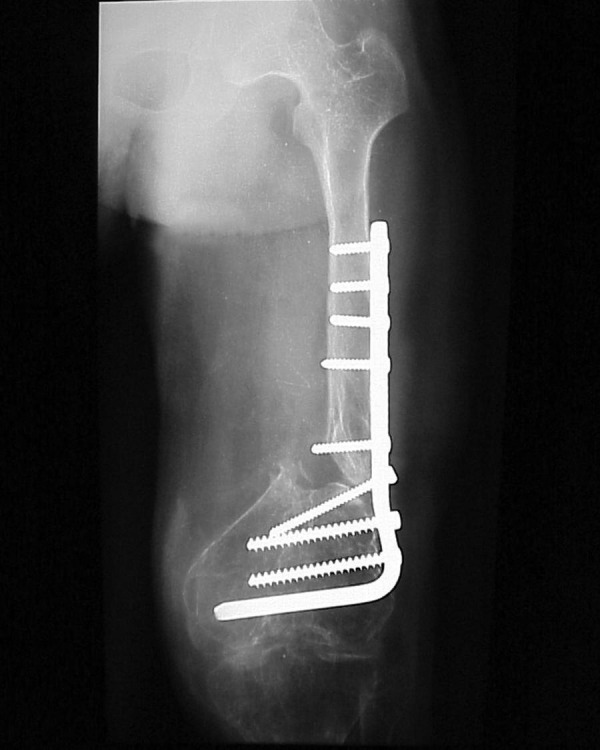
Post-operative Anterior-Posterior Radiograph Showing Approximation of Bone Ends, Condylar Plate Fixation and Iliac Bone Grafting
